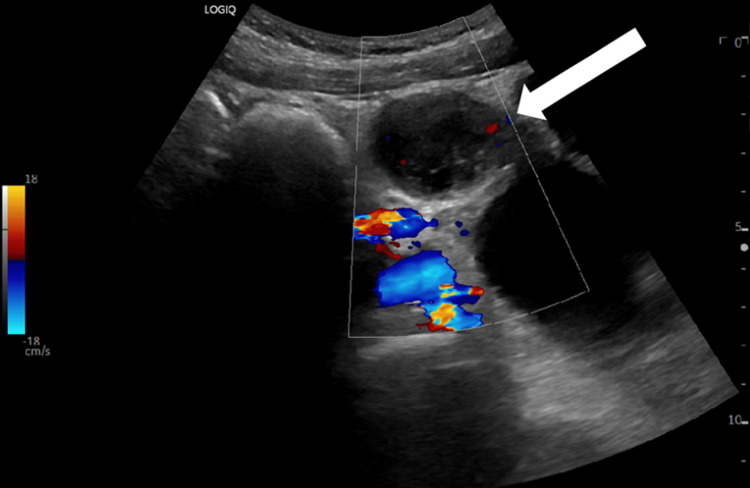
Longitudinal view of the left ovary with Doppler imaging.
